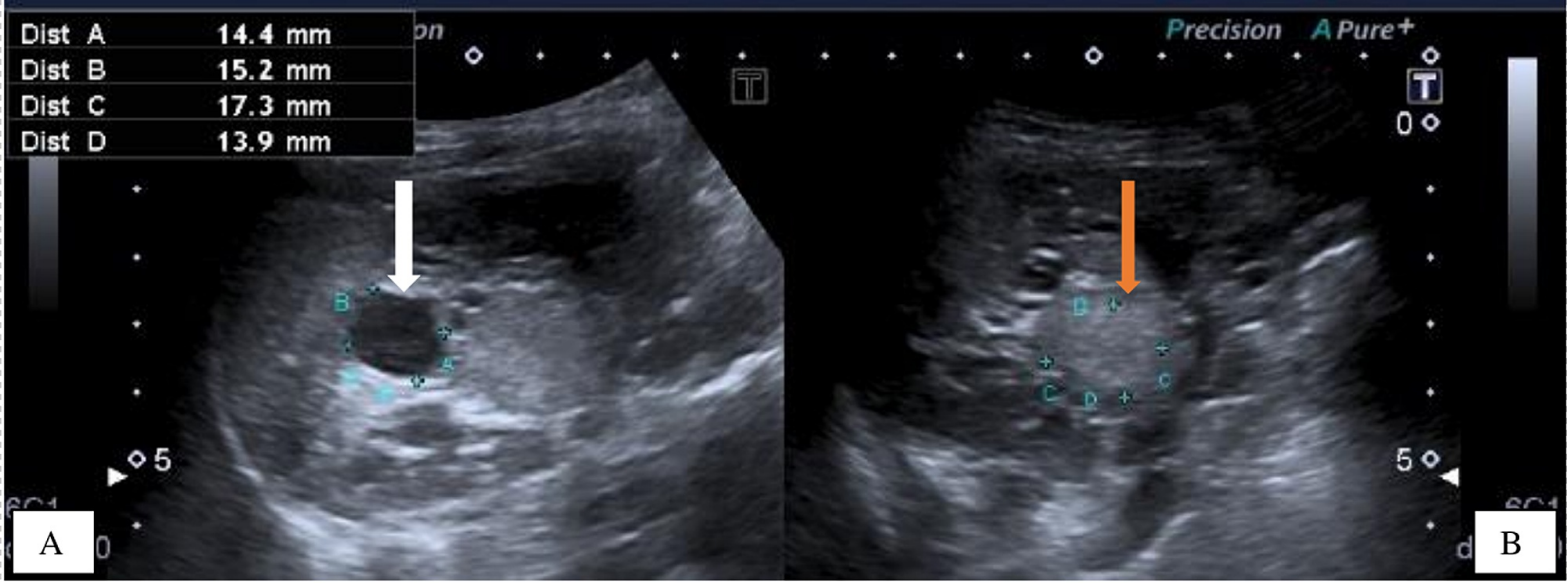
Ultrasound of the right kidney showing the simple renal cyst (white arrow) (8A) and the echogenic mass /angiomyolipoma (orange arrow) (8B) respectively.
